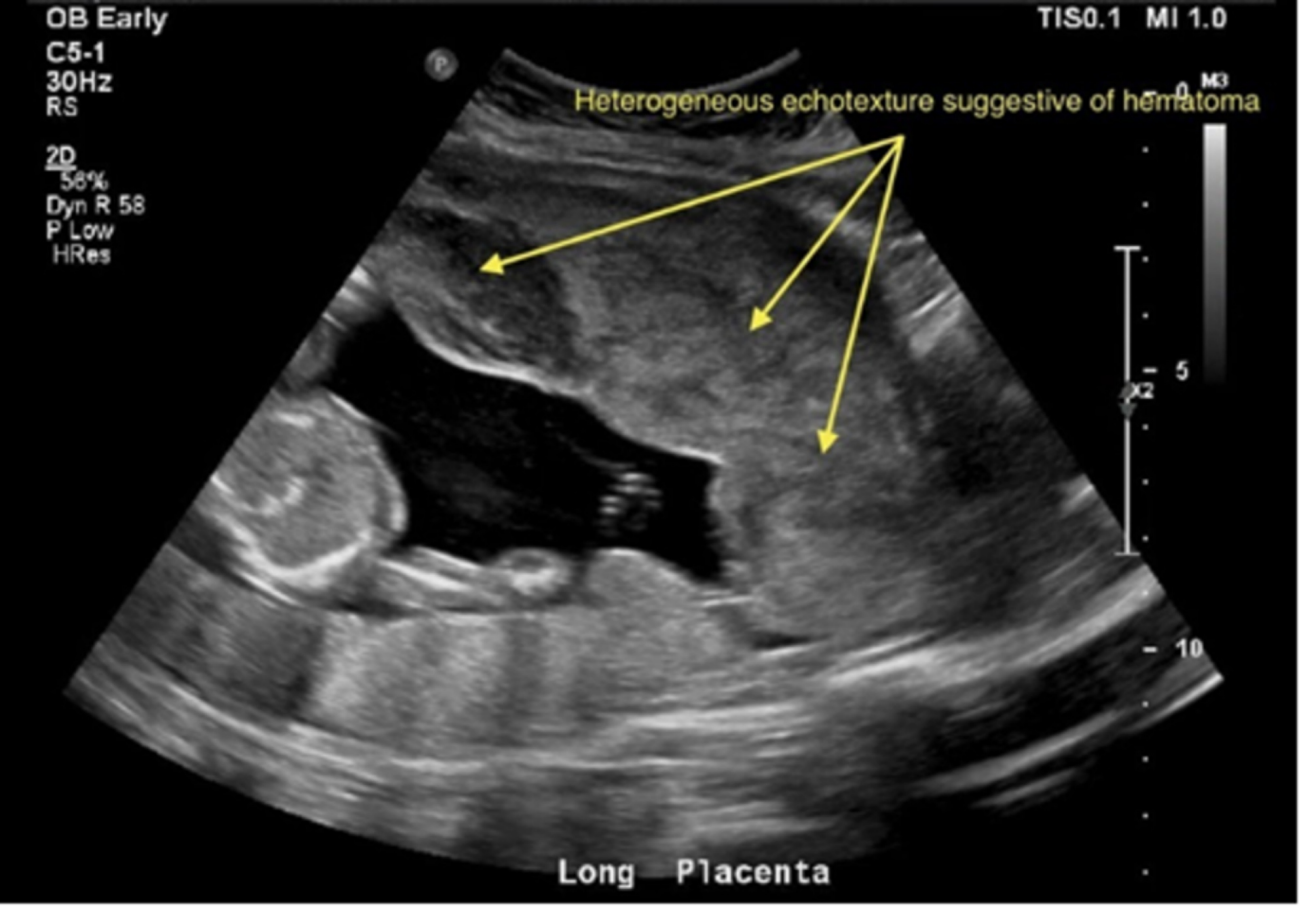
"U/S OB trauma limited" post motor vehicle collision, showing notable posterior placenta with a heterogeneous echotexture structure extending anteriorly U/S, ultrasound; OB, obstetric; findings were concerning for placental abruption.
